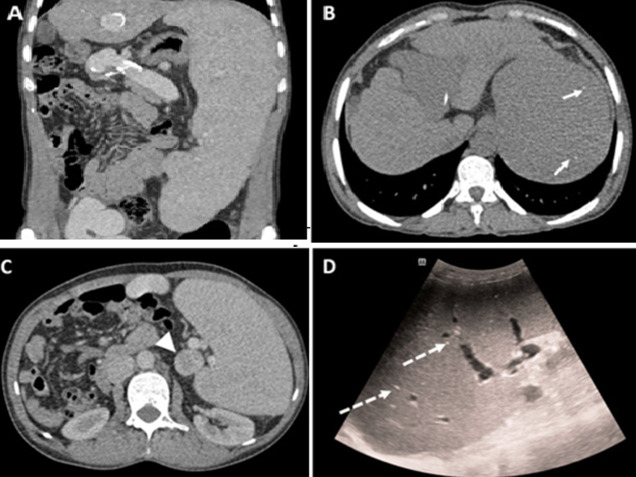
splenic parenchymal findings and accessory spleen identification: A) coronal; B,C) axial CT views along with ultrasound; D) massive splenomegaly (27cm) with multiple hyperdense foci (short arrows) consistent with Gamna-Gandy bodies; a peri-hilar nodular formation (triangle) resembling splenic tissue suggests an accessory spleen; hyperechoic foci with posterior acoustic shadowing are visible on ultrasound (dotted arrows)
